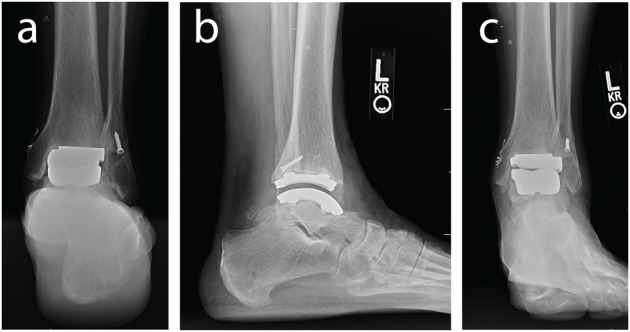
Weight bearing radiographs of the ankle and hindfoot with an implanted left TAR including: (a) hindfoot alignment view, (b) lateral view of the ankle, and (c) anterior-posterior view.
